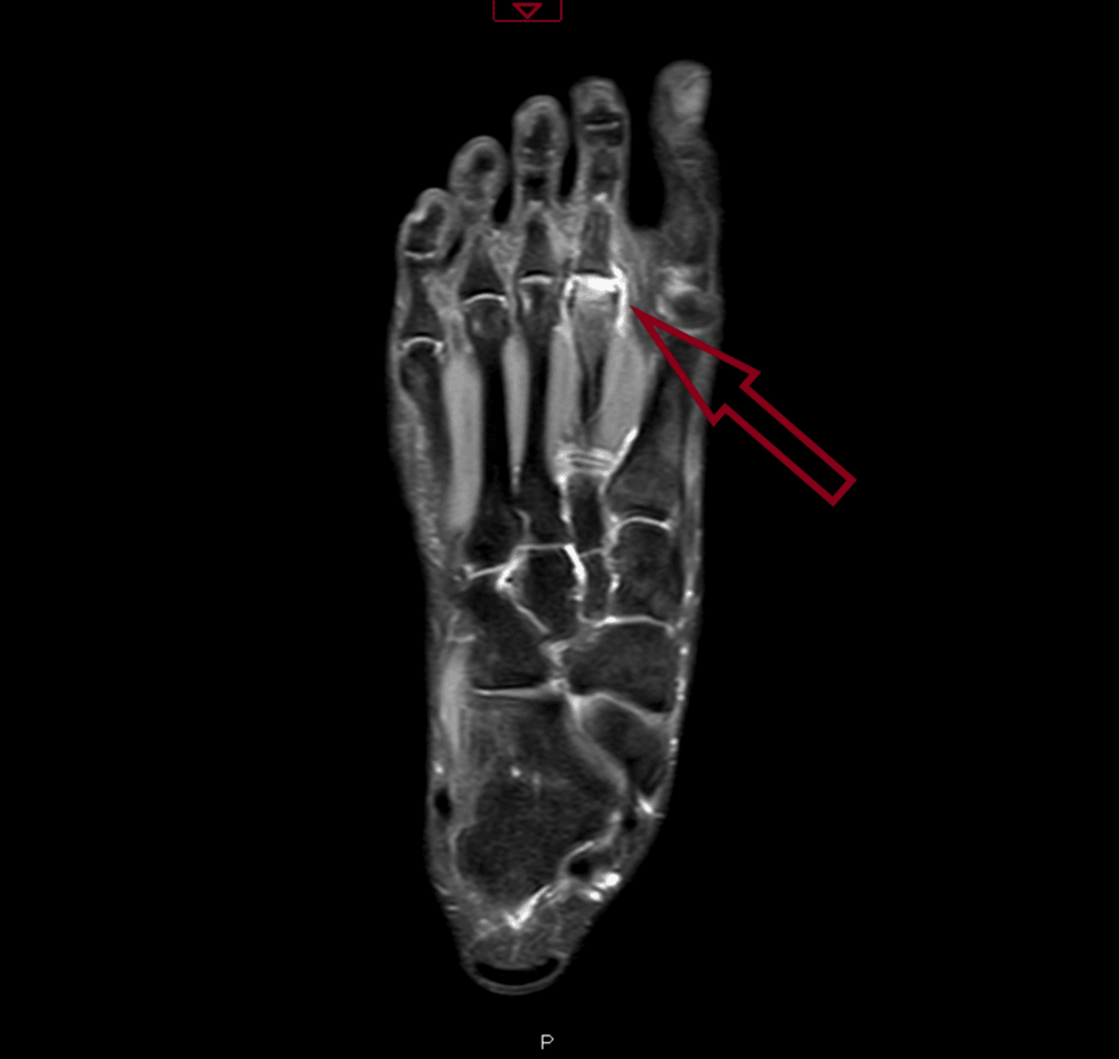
MRI scan showing destruction of the right second metatarsal and surrounding edema.
